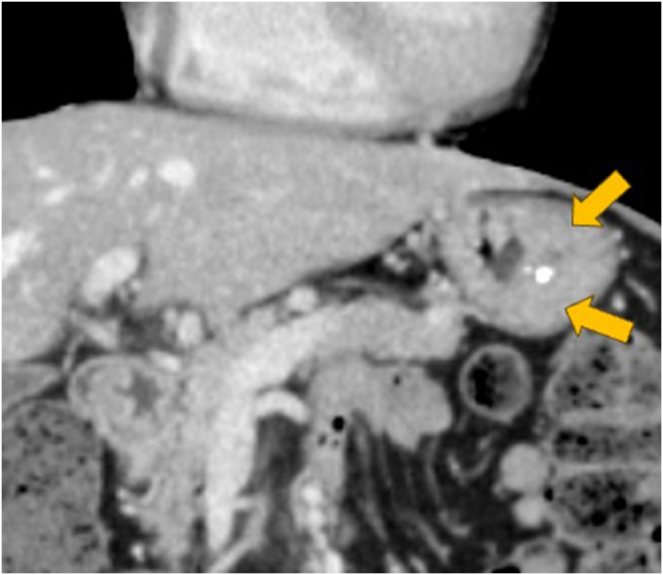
Abdominal computed tomography images show a submucosal tumor with calcification (left) and incremental contrast enhancement (right).
